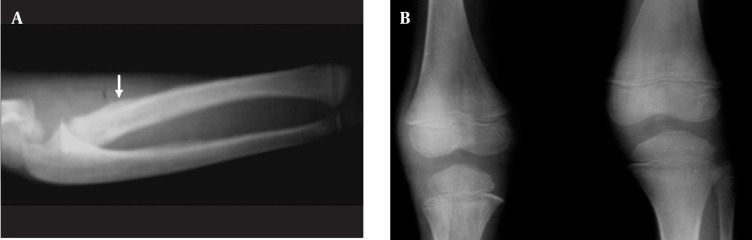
A 6-year-old girl with ALL presenting with pallor and right elbow pain A, Forearm X-Ray reveals periosteal reaction of radial proximal metaphysis with distal metaphyseal translucency(arrow);B, AP X-ray of knee joints reveals metaphyseal translucency in both distal femurs and proximal tibiae more prominent in the latter.
